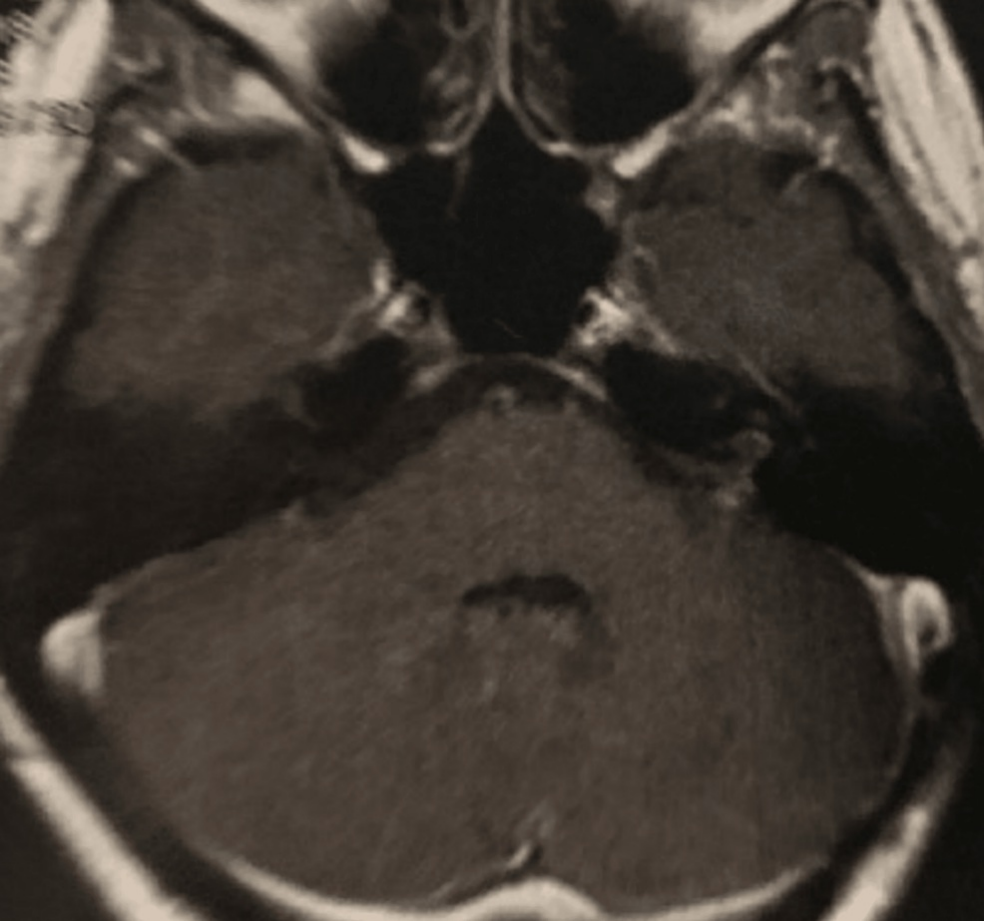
Postoperative axial contrast-enhanced T1-weighted MRI showed total resection
